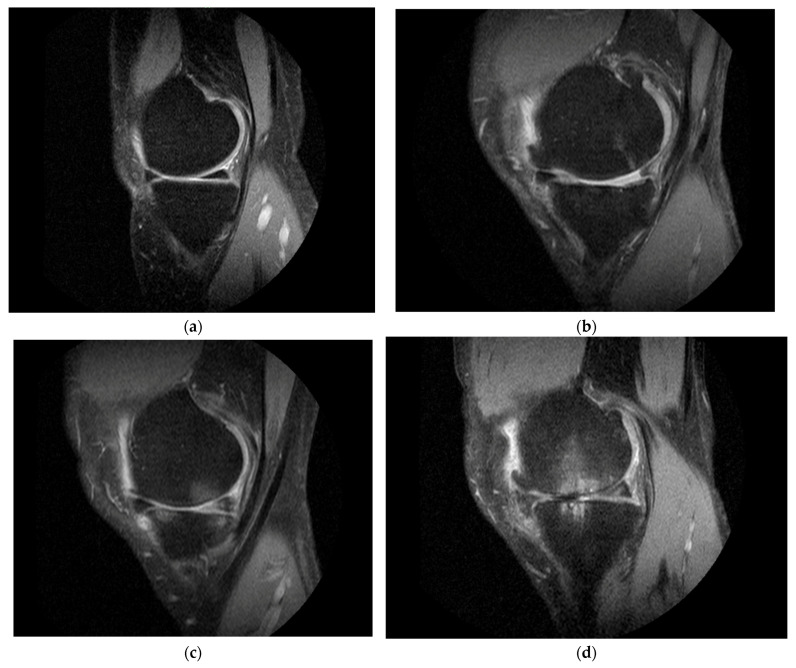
Representative T2-weighted MRI images showing bone marrow lesion grading in the knee. (a) Grade 0—No BML; (b) Grade 1; (c) Grade 2; (d) Grade 3.
